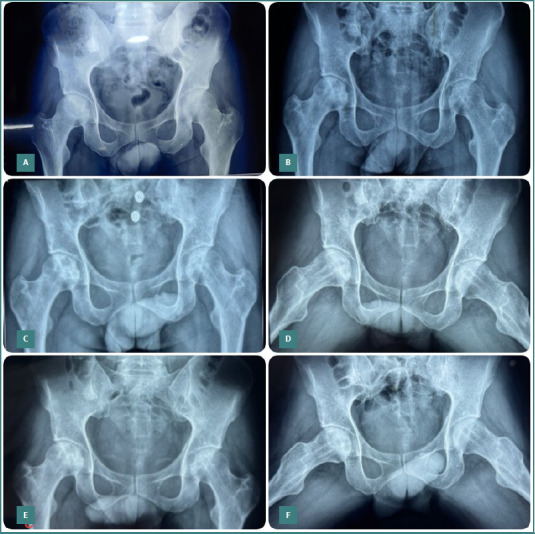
Serial anteroposterior pelvic radiographs showing bilateral hips of a patient with improved osteogenesis at the necrotic area and preservation of the spherical contour of the femoral head. A, Pre-operative radiograph; B, Six-month follow-up; C–D, Twelve-month follow-up; E–F, Twenty-four-month follow-up showing improved osteogenesis in the necrotic area and preservation of femoral head sphericity.
